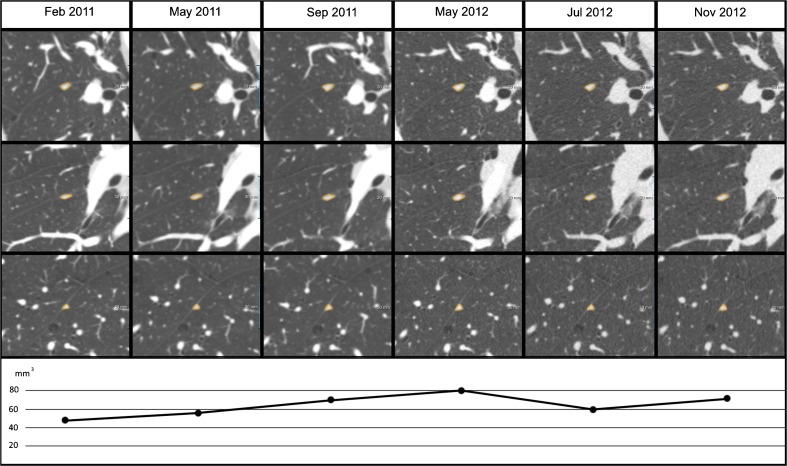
Example of growth in a perifissural nodule (PFN). A well-circumscribed, homogeneous, triangular-shaped solid nodule along the major fissure in the right lower lobe, presenting a PFN in an 58-year-old female without a prior malignancy. Nodule volume in the six consecutive CT scans ranged between 50 and 81 mm^3^ (diameter: 4.6–5.4) with a volume doubling time (VDT) of 361 days between CT2 and CT3, while a decrease with a VDT of -118 was seen between CT4 and CT5, as shown in the panels above
